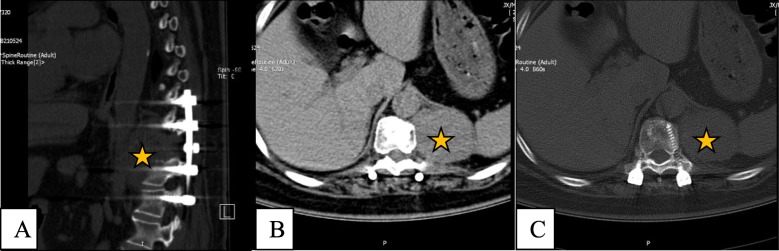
CT examination of the operative site (star indicated) 14 months after surgery. (a) CT sagittal view; (b) and (c) CT axial views
